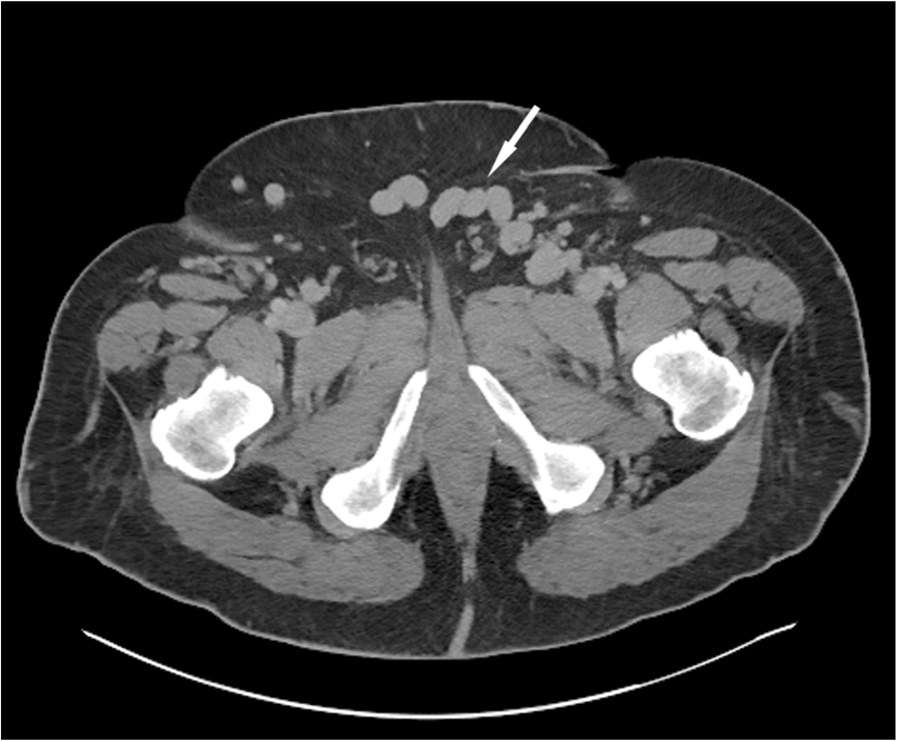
Axial CT of a 50-year old male presenting with bilateral lower extremity edema and pain. Torturous collaterals connecting the great saphenous veins immediately proximal to the saphenofemoral junctions are noted coursing through the anterior pelvic wall adipose tissue (white arrow)
